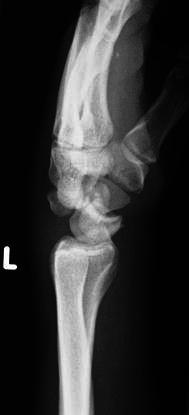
Wrist lateral X-ray showing volar perilunate dislocation
